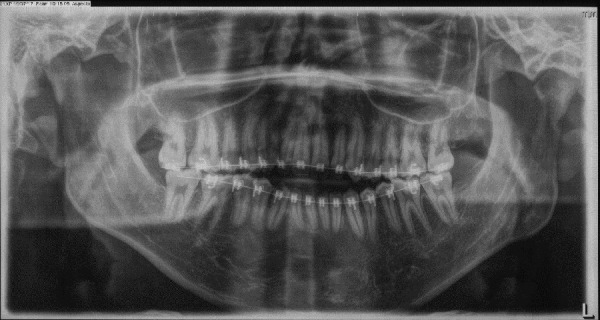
One-year panoramic radiograph follow up.
